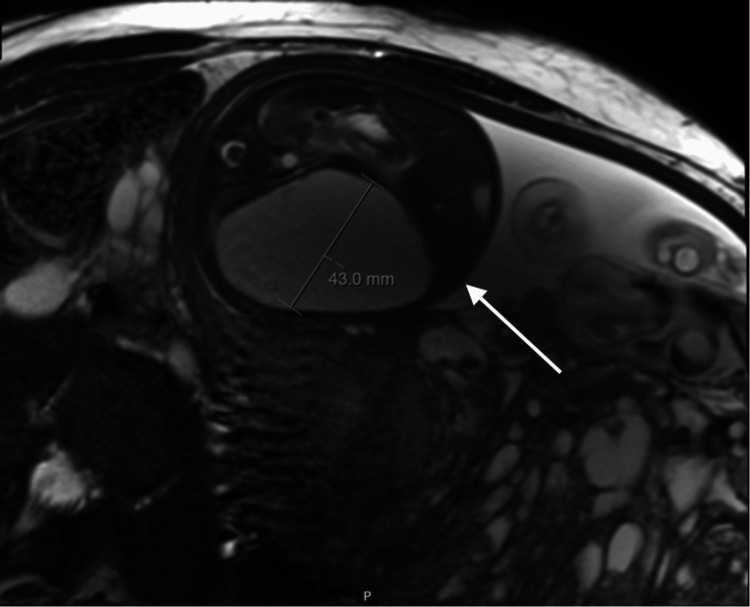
MRI at 28+0 weeks demonstrating an enlarging fetal liver cyst The arrow points to the fetal hepatic cyst seen on MRI
